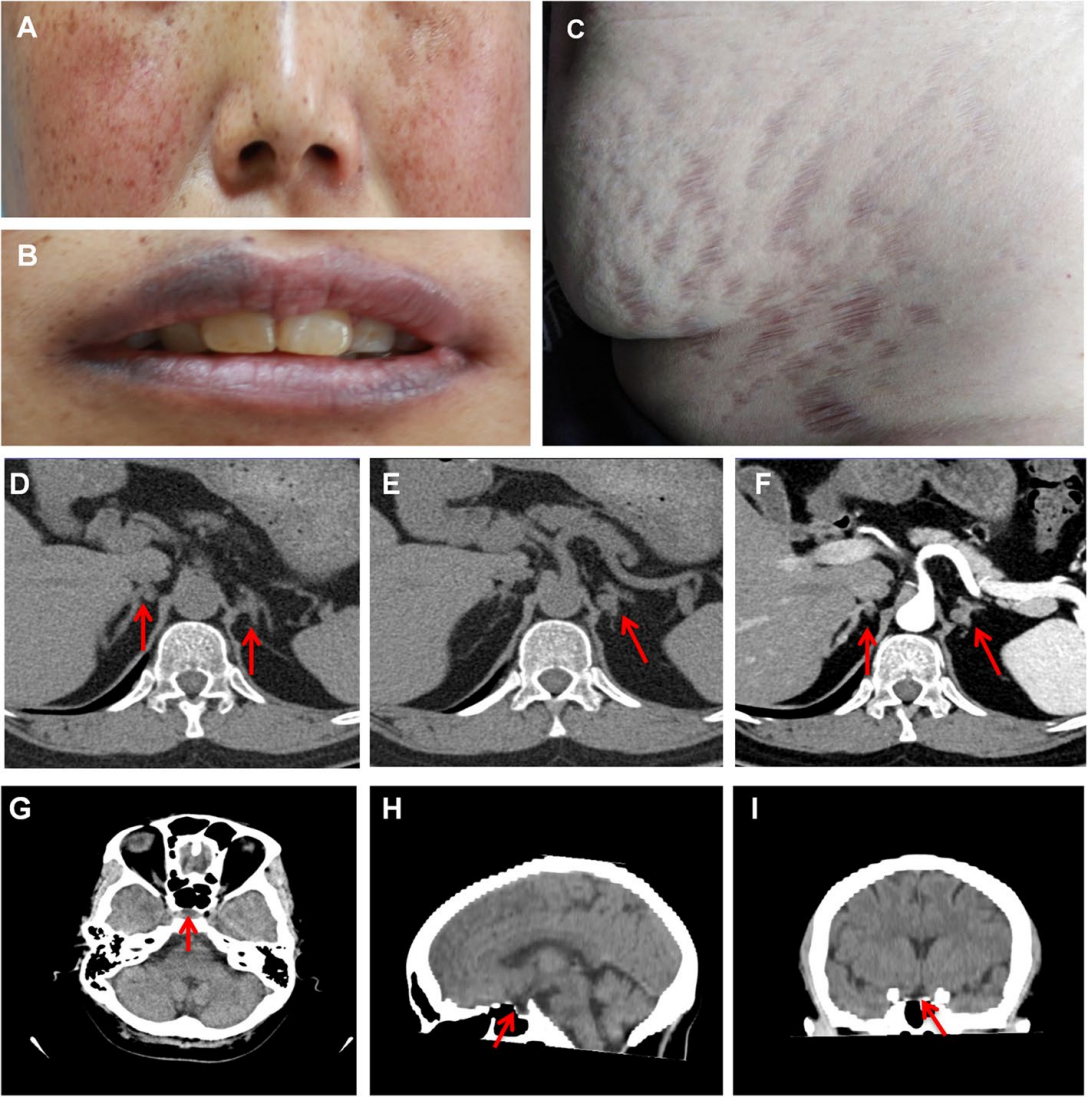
Clinical characteristics and imaging of the patient. (A) Mild facial plethora, pigmented spots on the face; (B) Pigmented spots on lips; (C) Purple striae on the lower abdomen. (D-F) Adrenal computed tomography (CT) showed multiple small nodules on both sides of the adrenal glands (the larger diameter is 8 mm), considered adenomas; (G-I) Brain CT showed the ischaemic focus of the left half oval centre and the partial empty sella
